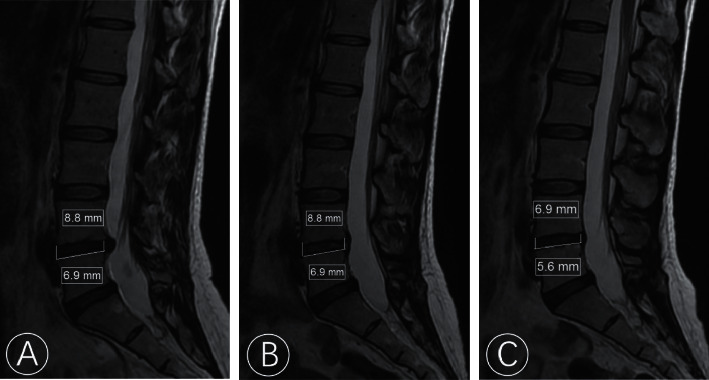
Comparison of disc height (DH) on MRI preoperatively (a), at 3 days (b), and at 1 year after operation (c) with the upper number which represents the anterior DH and the lower number represents the posterior DH.
